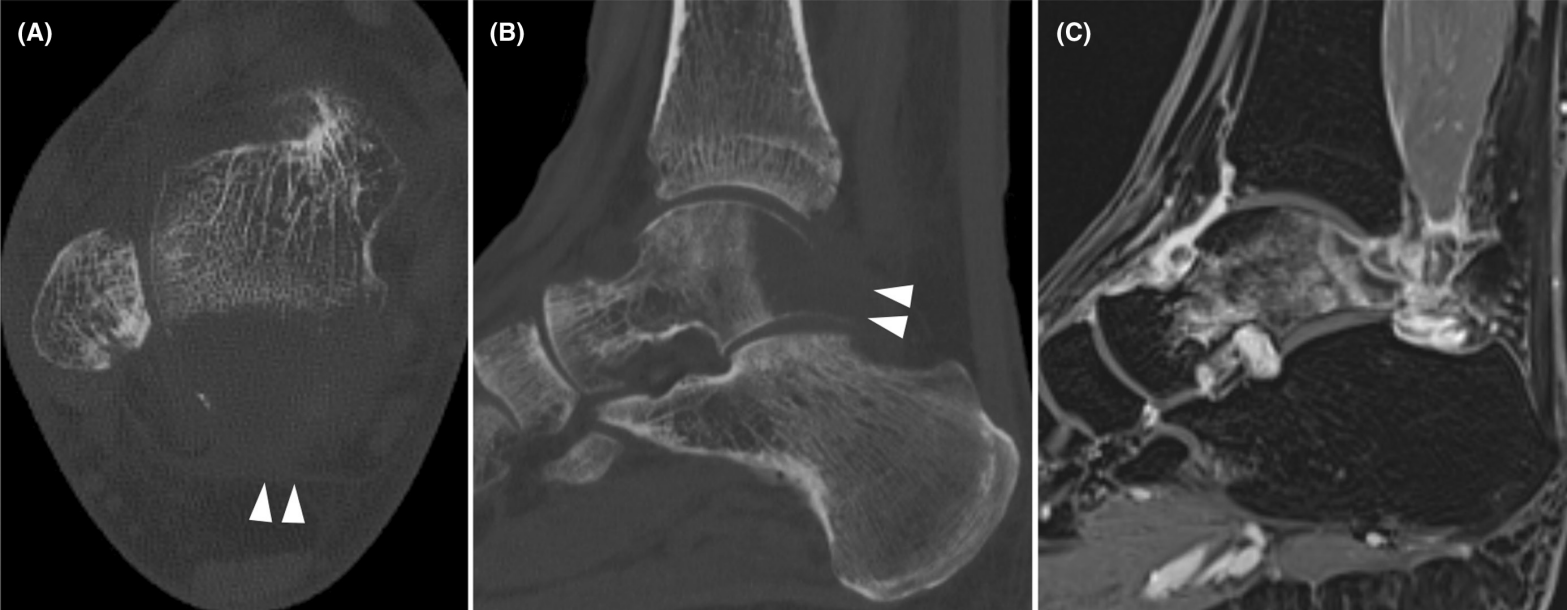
Preoperative ankle computed tomography: axial (A) and sagittal (B). The white arrows indicate the osteolytic lesion. Preoperative ankle magnetic resonance imaging: sagittal (C).
